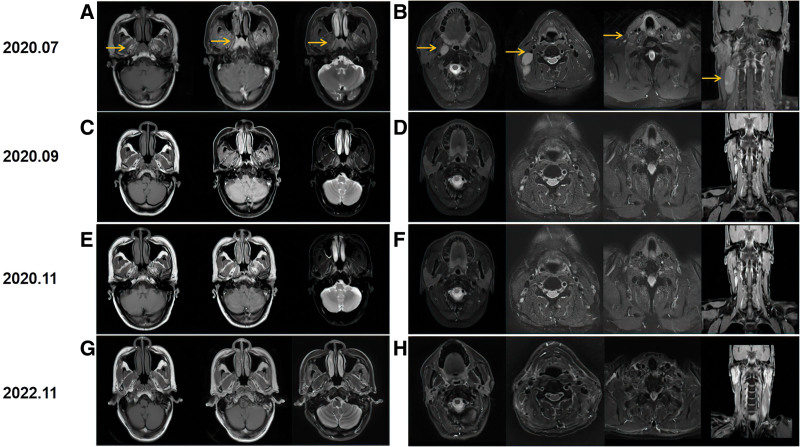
(A and B) MRI of the nasopharynx and lymph node before treatment (August, 2020). (C and D) MRI of the nasopharynx and lymph node after 2 cycles of treatment (September, 2020). (E and F) MRI of the nasopharynx and lymph node after 4 cycles of treatment (November, 2020). (G and H) MRI of the nasopharynx and lymph node about 24 months after treatment (November, 2022). MRI = magnetic resonance imaging.
